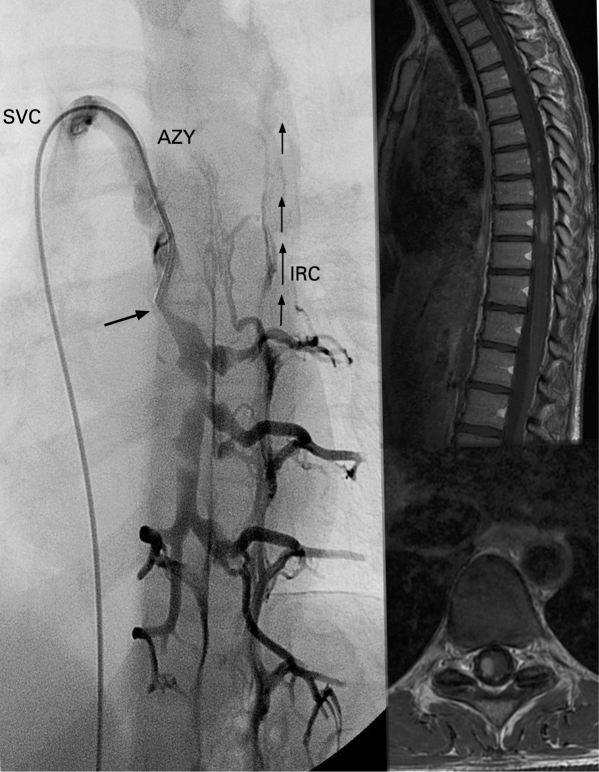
Selective venography and MRI in a clinically defined multiple sclerosis case with chronic cerebrospinal venous insufficiency pattern D. Left: selective venography showing membranous obstruction of the outlet of the azygous vein (AZY) combined with atresia of the descending azygous vein (arrow). Due to multilevel obstruction of the azygous system, the vertebral plexus is dilated below the atresia, and the blood is drained through intrarachidian collateral circles (IRC) in an upward direction. Top right: sagittal T1 weighted imaging after gadolinium injection of the same case, showing typical multiple sclerosis lesions of the spinal cord. The enhanced gadolinium image shows intrarachidian venous plexuses in the form of small spots. Bottom right: axial merge T2 of the same patient at the cervical level with dilated extrarachidian venous plexuses. SVC, superior vena cava.
